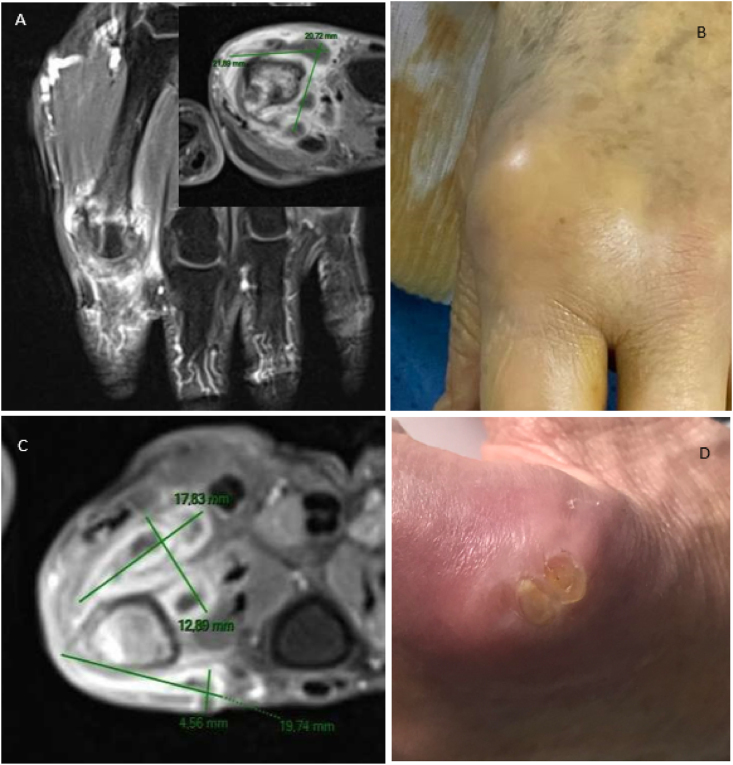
MRI and clinical evolution of *C. blankii* osteoarticular infection. A. MRI at D140: Osteoarthritis of the metacarpophalangeal joint of the second ray with an abscess in the adjacent soft tissues, measuring approximately 2x2x2 cm, and probable phlegmon around the extensor tendon sheath of the second ray. B. D150 before puncture: swelling of the second metacarpophalangeal joint, suggestive of synovitis and/or arthritis. C. MRI at D232: Extensive intra-articular collection measuring 18 × 13 mm on the palmar side and on the dorsal side measuring 19 × 5 mm (green lines). D. Clinical evolution at D243: Erythematous peri-articular area, seeping opening related to a fistula. (For interpretation of the references to colour in this figure legend, the reader is referred to the Web version of this article.)
